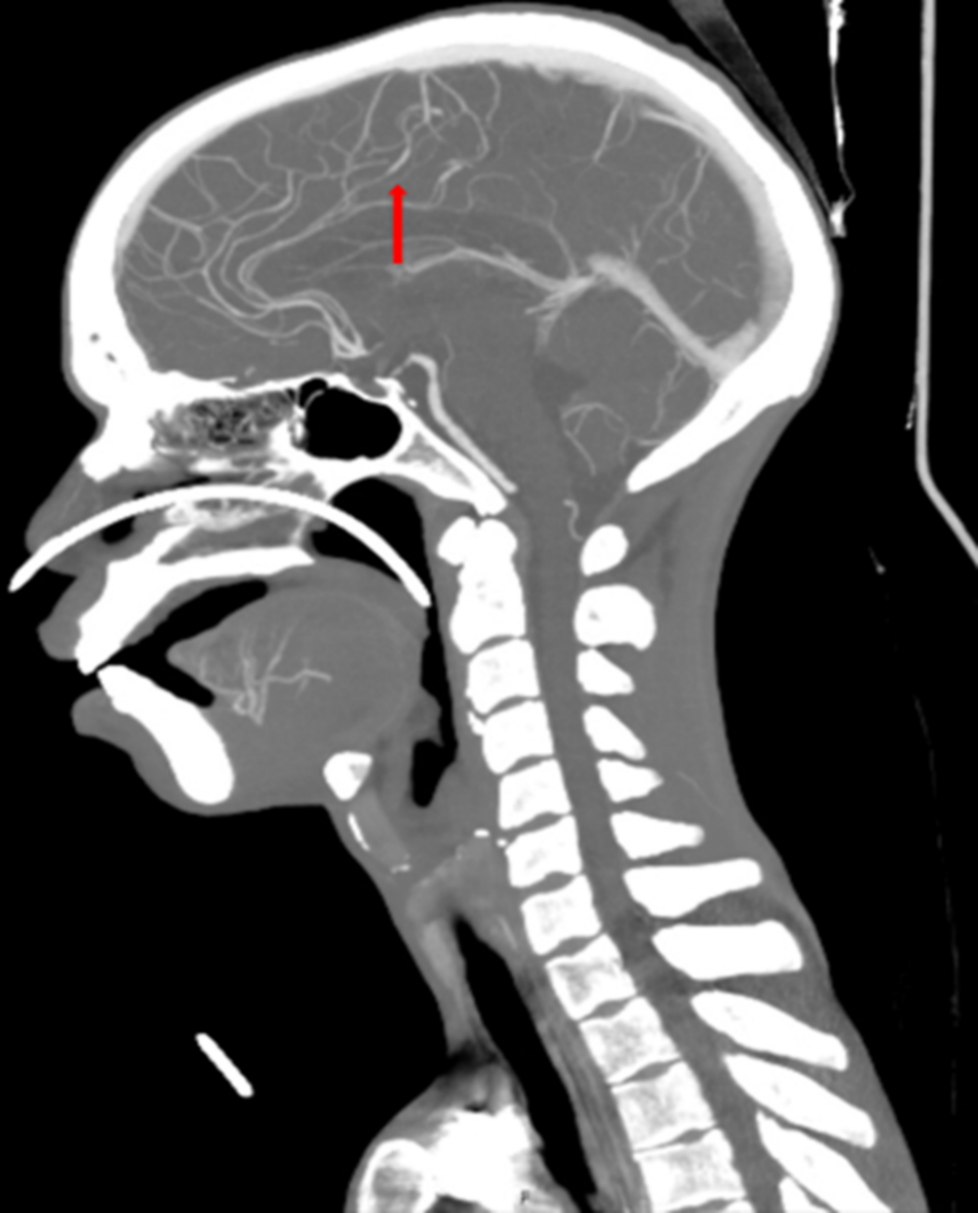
CTA of the head and neck The image shows multifocal areas of mild to moderate stenosis (red arrow) CTA: computed tomography angiography
